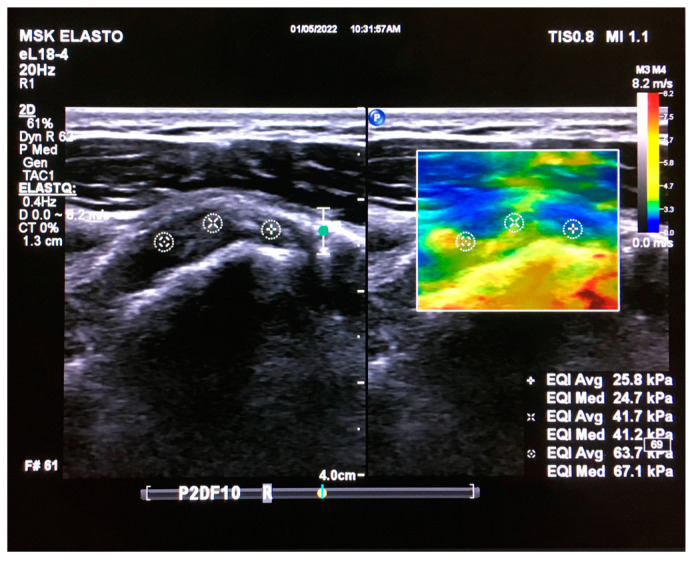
Coronal real-time ultrasound elastography image of the supraspinatus tendon in a healthy young adult. B-mode ultrasound image (left) and ultrasound elastogram (right). The elasticity was measured at the proximal (+), middle (×), and myotendinous (*) parts of the tendon. A circular ROI with a diameter of 5 mm was manually applied to measure the tendon’s elasticity. Each measurement included the average and median of the elasticity of this circular ROI of the tendon in the rectangular ROI (which varied in size), focusing on the central part of the tendon. The image was obtained from the University of Washington Medical Imaging database with the required permissions.
